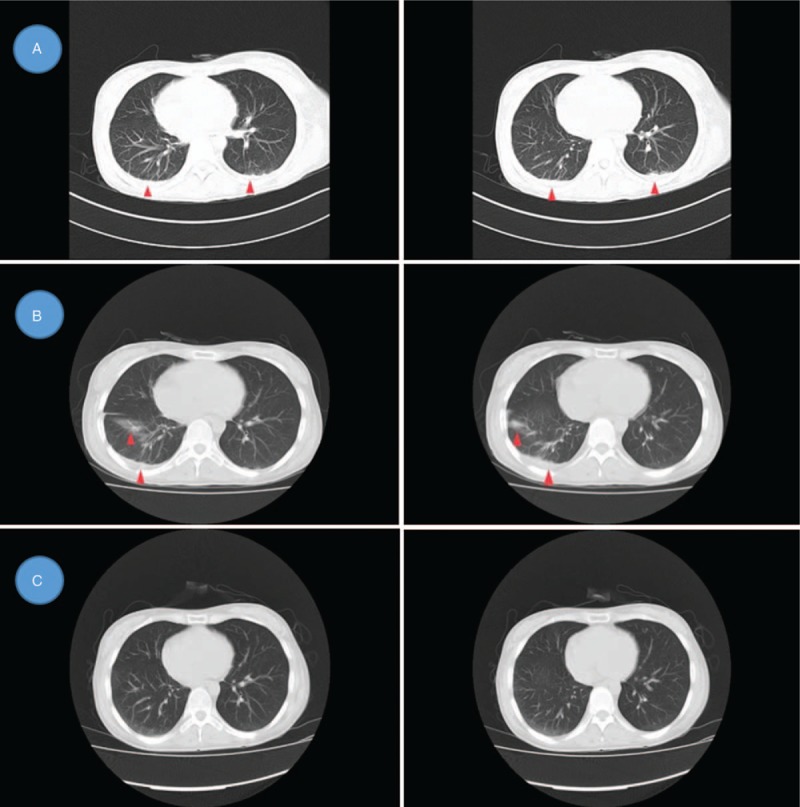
(A) Lung CT scan detected the bilateral pleural effusion. (B) On day 23, lung CT was reviewed: the right lower lobe had new inflammation, the right pleural effusion increased more than before. (C) The lung CT was reviewed before discharge and the lung infection was eliminated. CT, computed tomography.
